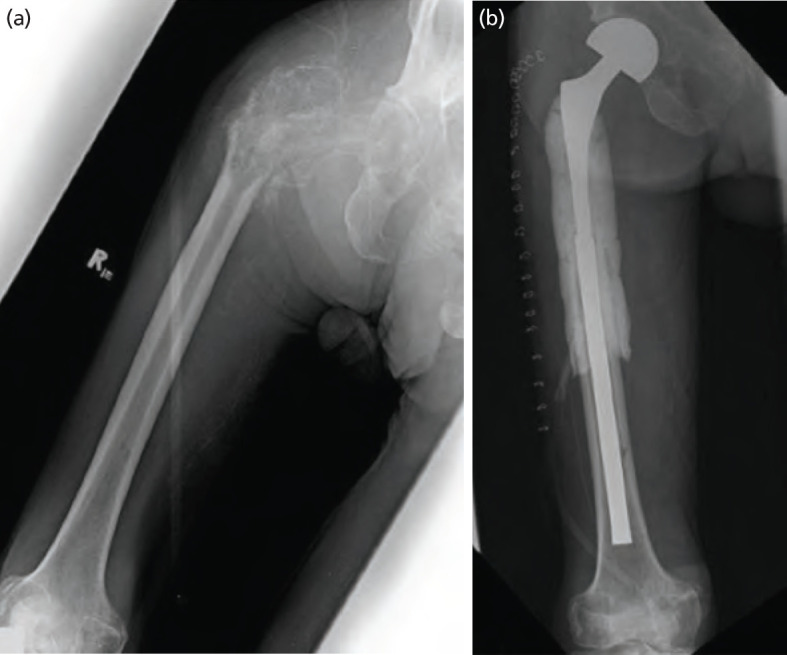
(a) Injury films of the right femur in AP view, revealing a fracture and a lytic lesion in the per trochanteric area of the right proximal femur. (b) Post-operative radiograph at one year and two months, with no signs of dislocation, recurrence, or implant failure.
